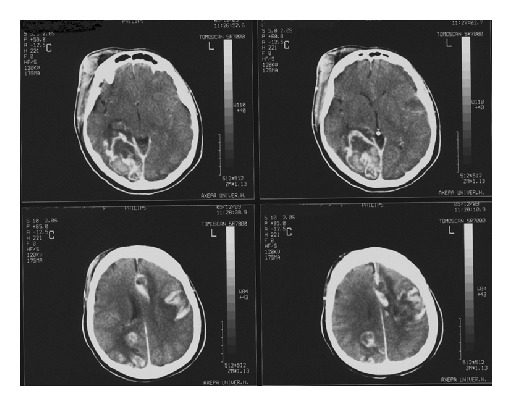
Brain CT scan with hemorrhagic transformation of infarcts.
